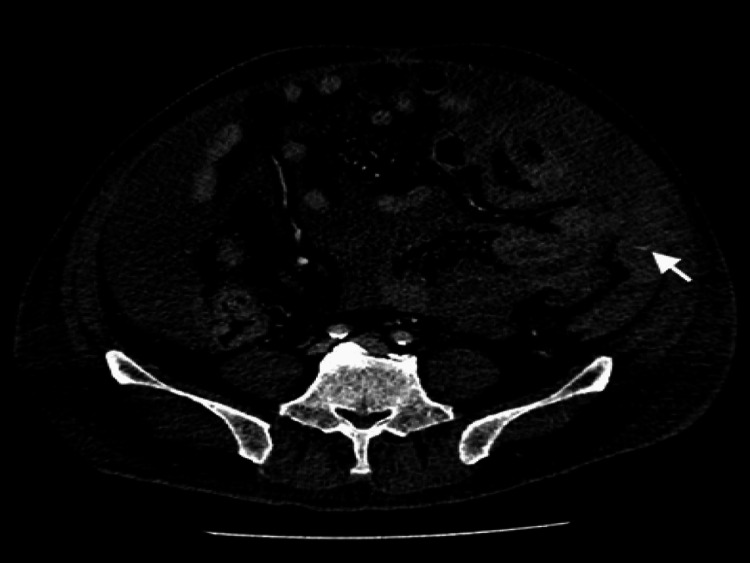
CT lower abdomen - pelvis (venous phase) image showing extravasation of contrast material (white arrow) within a hematoma in the left paracolic gutter, along the course of the deep circumflex iliac artery.
